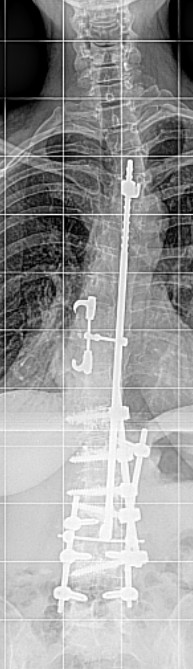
X-ray of the spine in anterior-posterior projection one year after additional ventral augmentation
